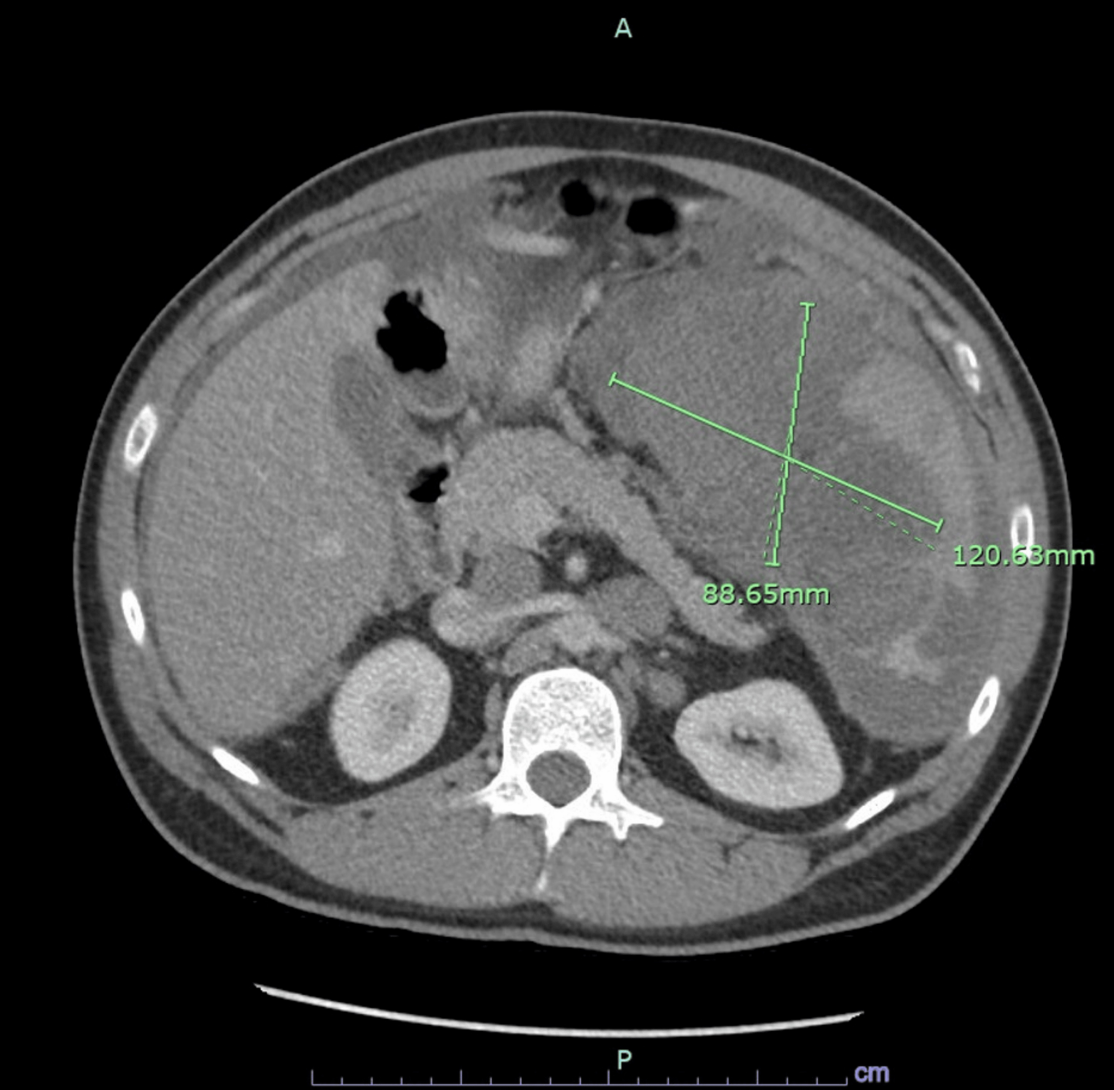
Initial computed tomography of abdomen and pelvis with contrast showing extensive focal and confluent lesions throughout the spleen with largest confluent mass measuring 12.1 cm.
